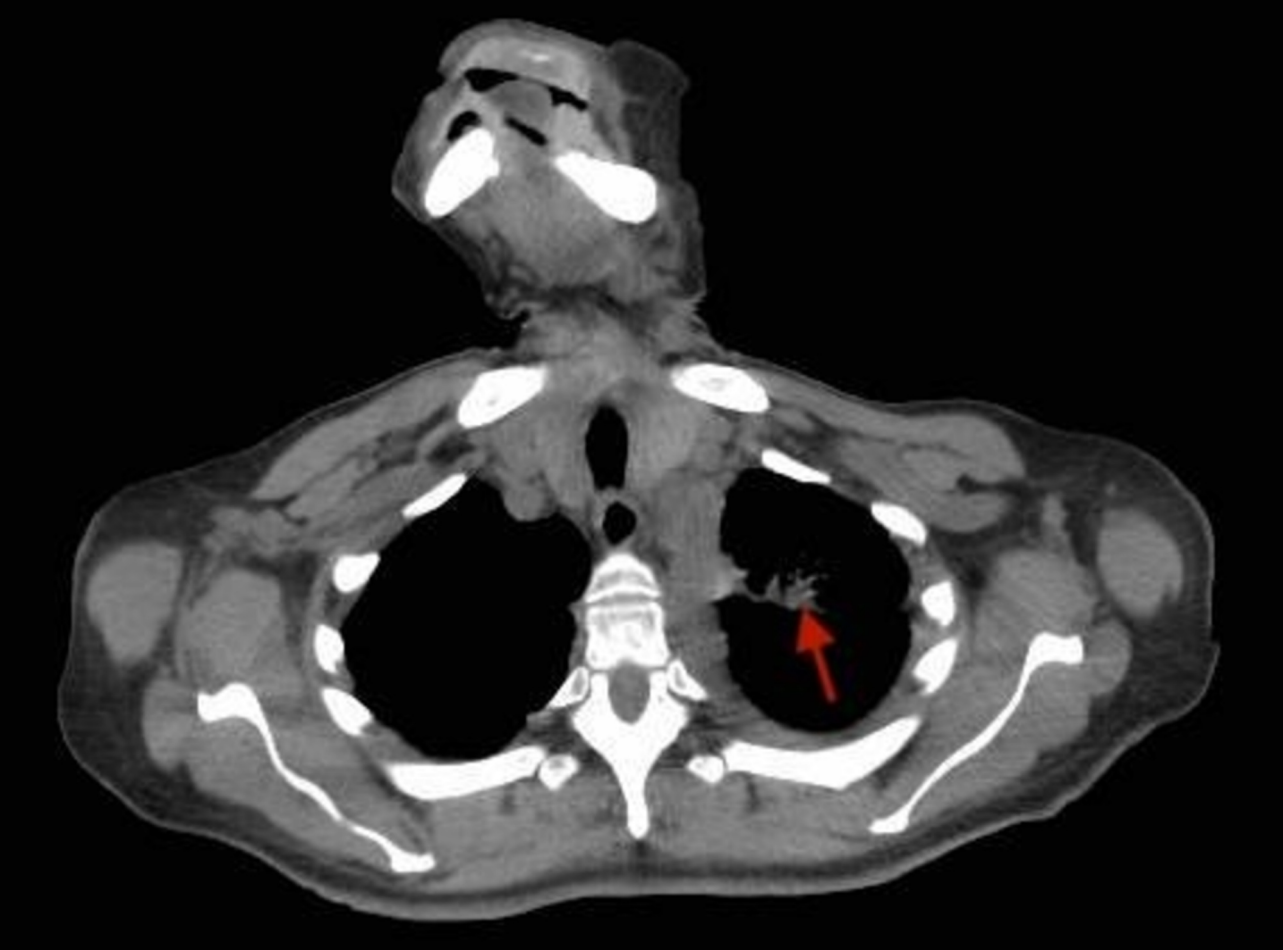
Axial CECT (contrast-enhanced computed tomography) scan of the chest showing a left lung mass extending into the upper lobe (red arrow).
